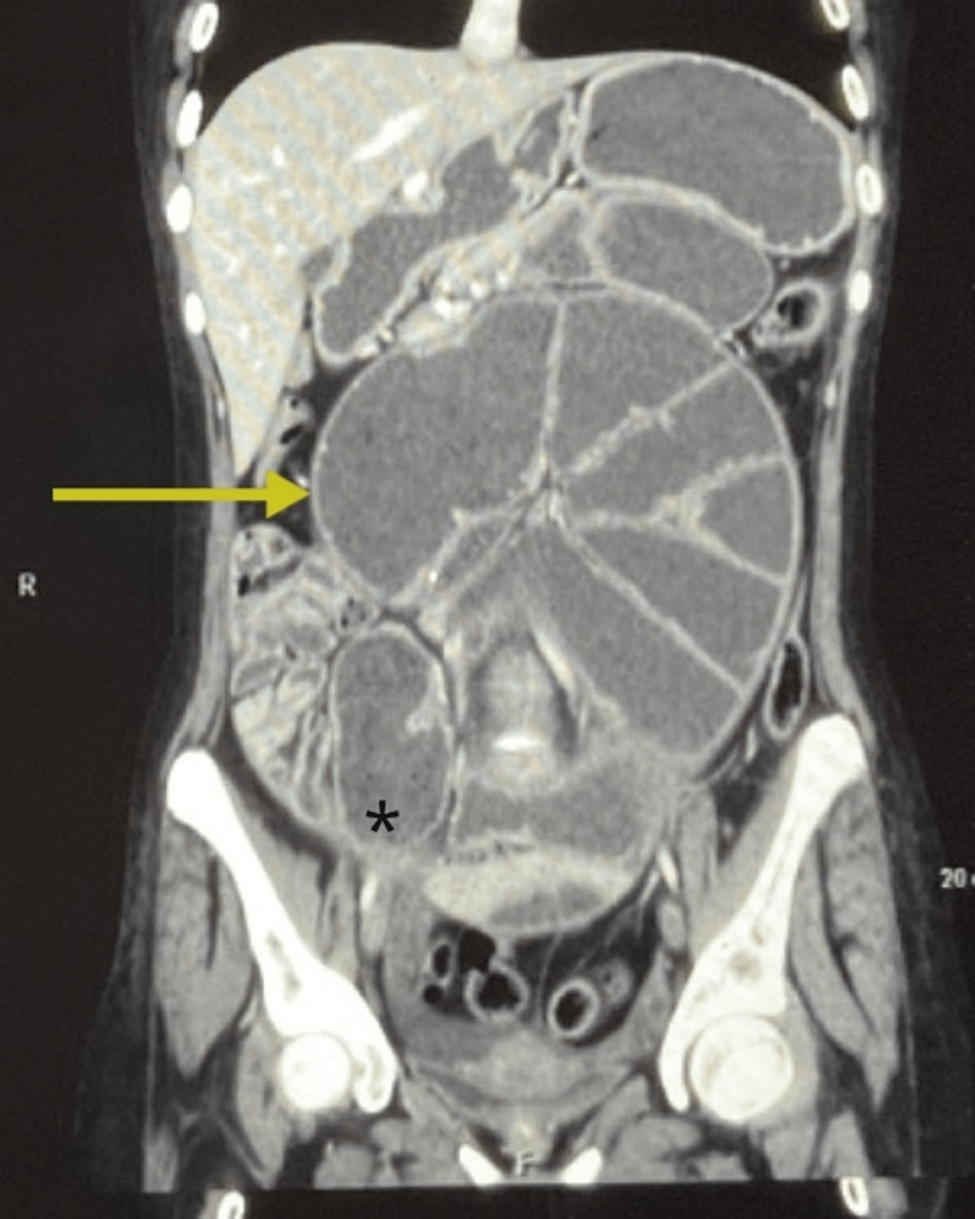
CECT abdomen - coronal section Circumferential homogenously enhancing long segment (single arrow) (4.4 cm) wall thickening (7.3 mm) suggestive of small bowel stricture seen in the proximal ileal loop. A similar appearing stricture is seen in the mid-ileal loops as well (3 cm in length and 7 mm in thickness- double arrow). Jejunal loops are grossly dilated (6.2 cm) with multiple air-fluid levels and are seen in the central abdomen. The stomach and duodenum also appear distended. There is the presence of collapsed ileal loops lateral and posterior to the caecum (marked with *). CECT - contrast-enhanced computed tomography
